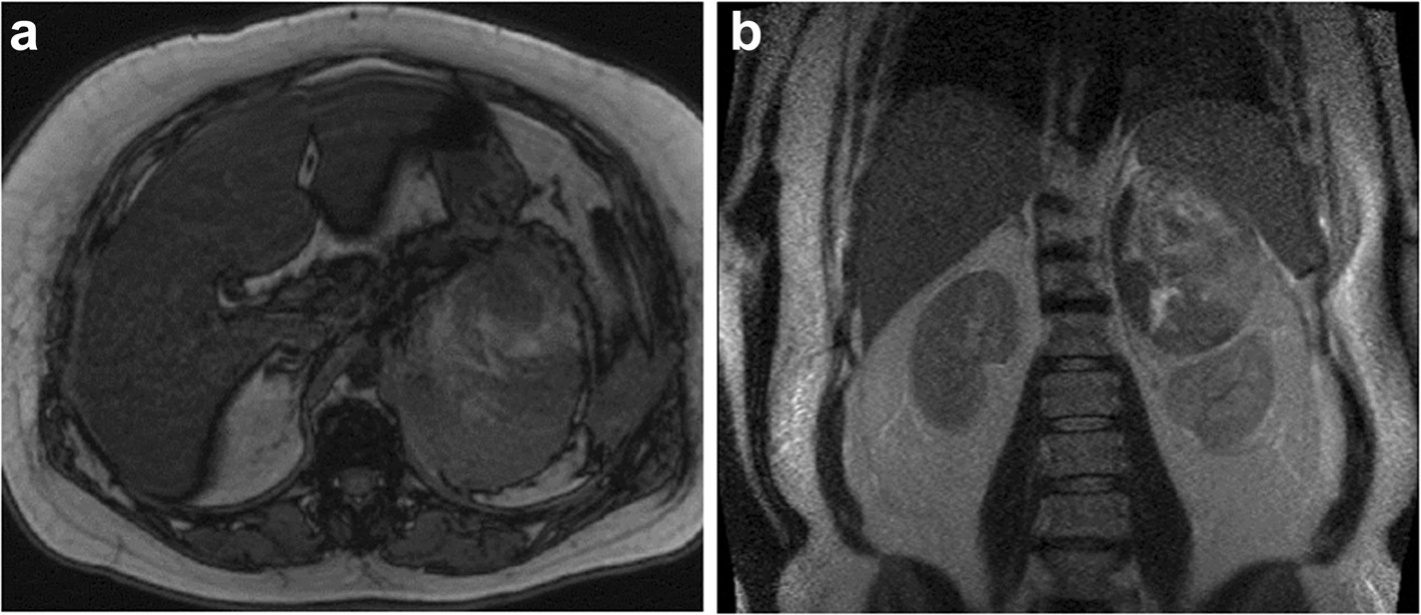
MRI images findings. a. A tumor exhibiting non-uniform high to low signals on both T1 and T2 MRI images was observed in the left adrenal gland (Transverse plane). b. Coronal plane.
